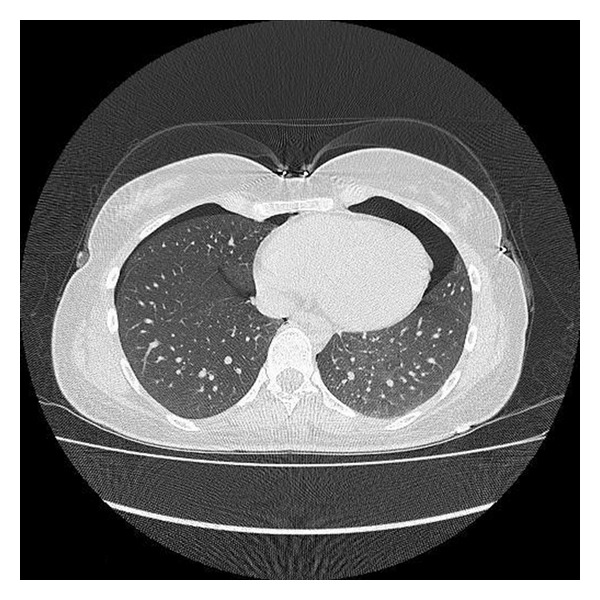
Computed tomography scan shows bilateral pneumothoraces.
